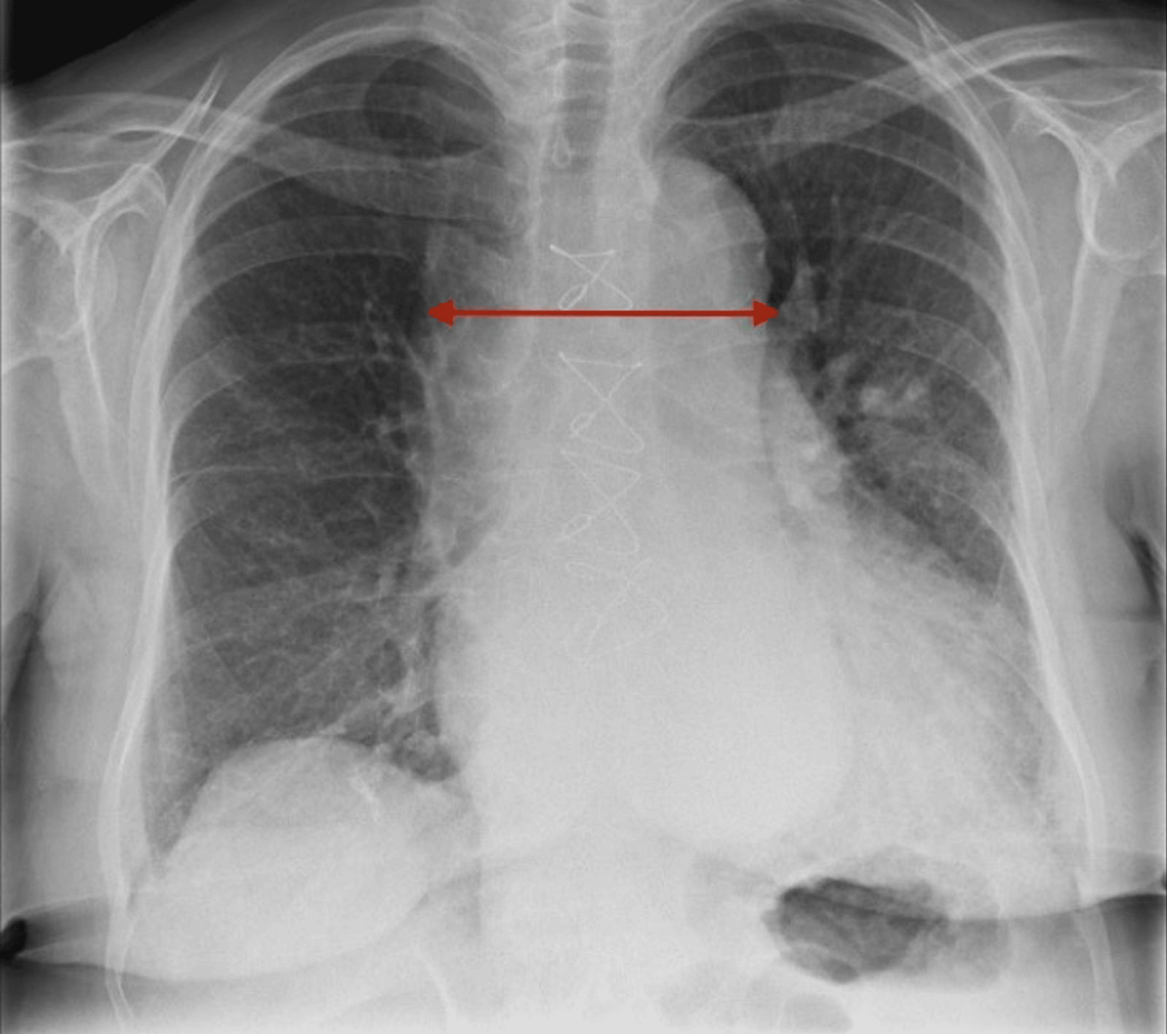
Chest X-ray (CXR) The red line on the CXR shows the widened mediastinum.
